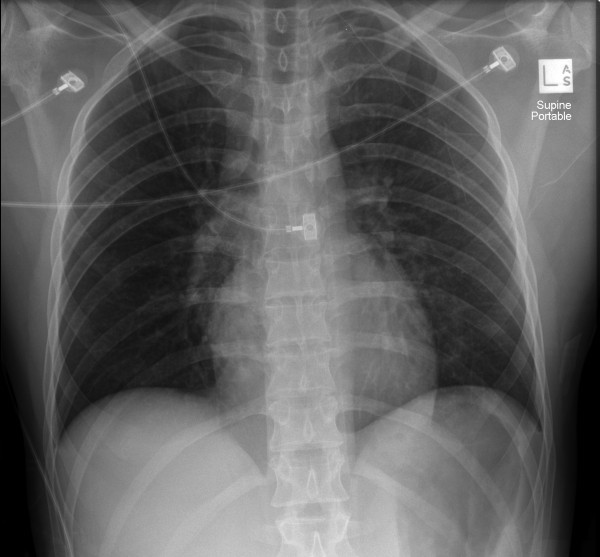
Initial anteroposterior chest X-ray.
